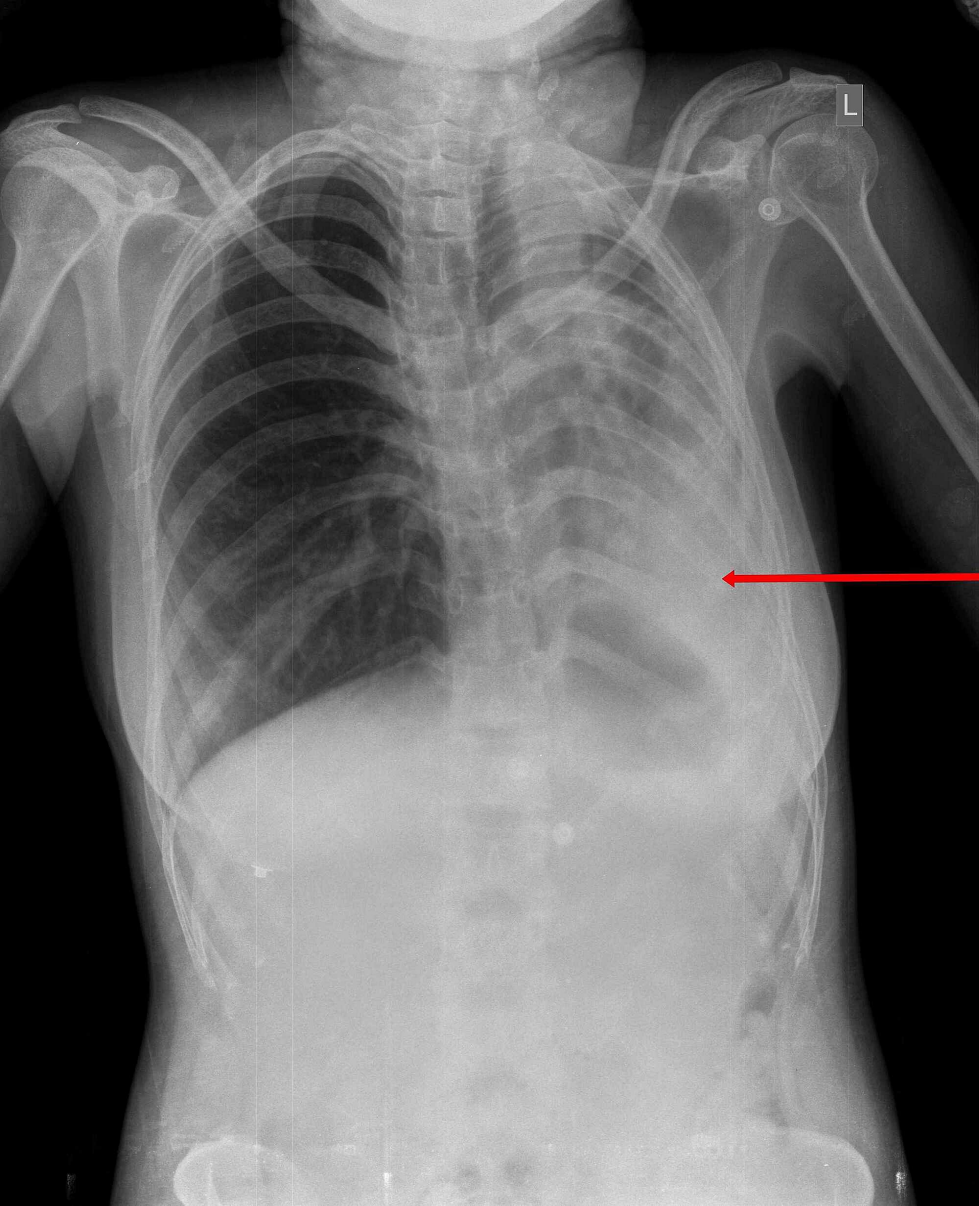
Chest radiograph, posteroanterior view, showing unilateral left side post tuberculosis fibrosis
